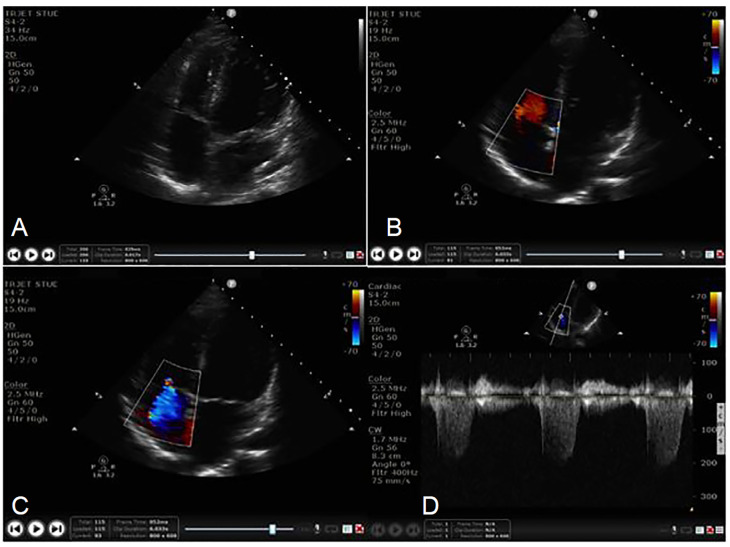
Tricuspid regurgitant jet velocity image elements. 2A. Apical 4-Chamber, 2B. Color box positioning, 2C. Tricuspid regurgitant jet signal optimization, 2D. Continuous-wave Doppler interrogation.
